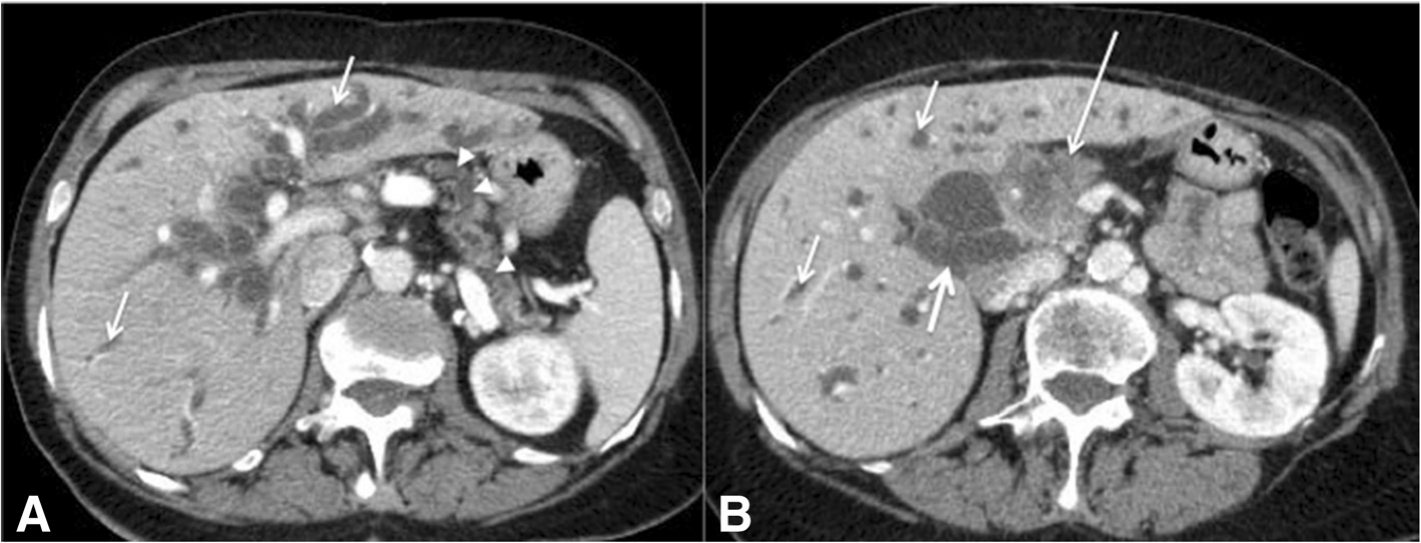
A 58-year-old man with biliary obstruction caused by adenocarcinoma of the pancreas. Axial (A) and (B) CT images of the abdomen obtained after intravenous contrast administration demonstrating dilatation of the intra- (thin arrows) and extrahepatic bile ducts (thick arrow), a pancreatic head mass (adenocarcinoma) with irregular contours and poorly defined borders (long arrow), and dilatation of the pancreatic duct (arrowheads).
